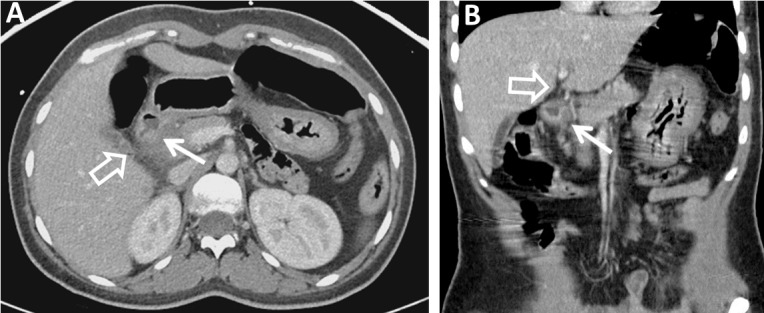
Contrast-enhanced Axial (A) and Coronal (B) CT Images of the Abdomen and Pelvis (Post-Treatment) Contrast-enhanced axial (A) and coronal (B) CT images of the abdomen and pelvis two weeks after conservative treatment demonstrate a small duodenal diverticulum with significantly improved surrounding inflammatory changes (arrows). Intrahepatic biliary ductal dilation is significantly improved (open arrow).
